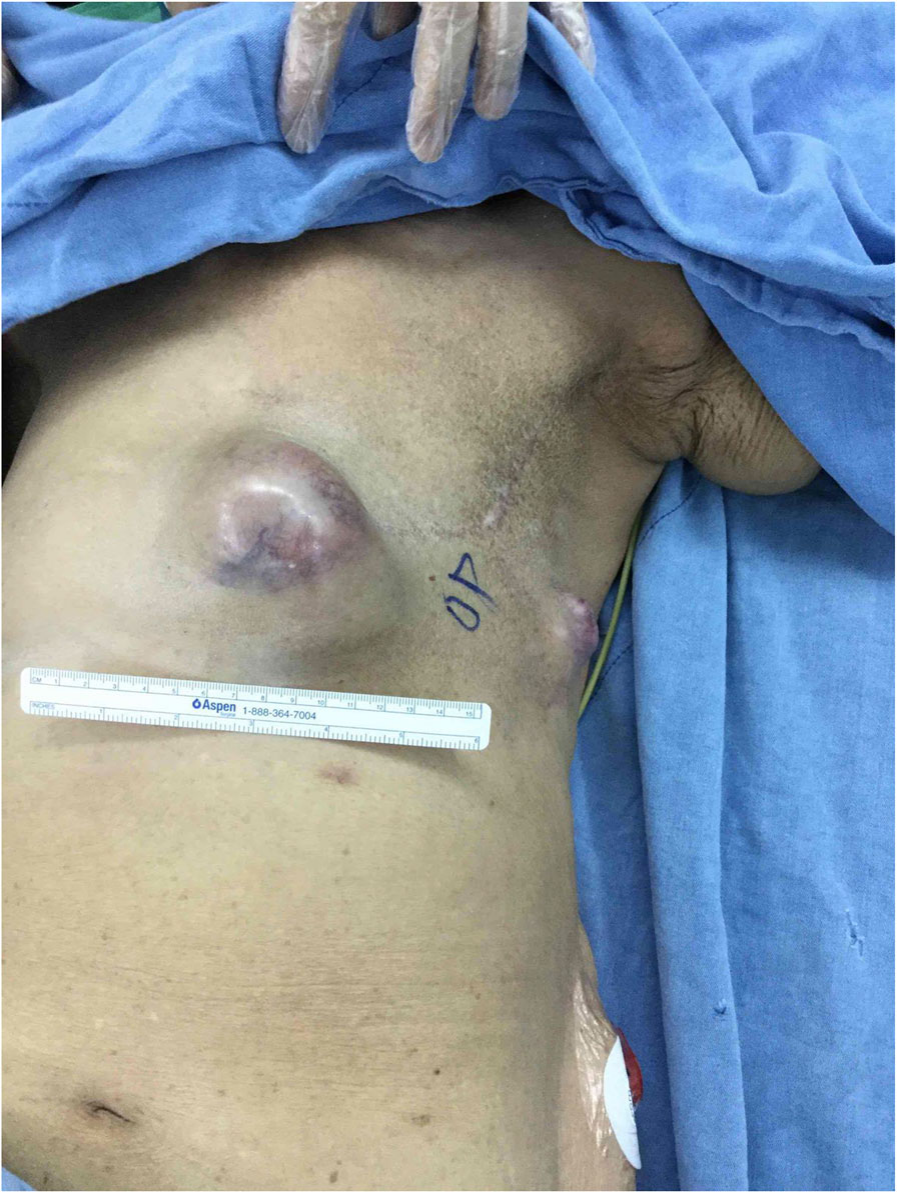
Showed recurrent tumors over left parasternal region and left sub-axillary region
